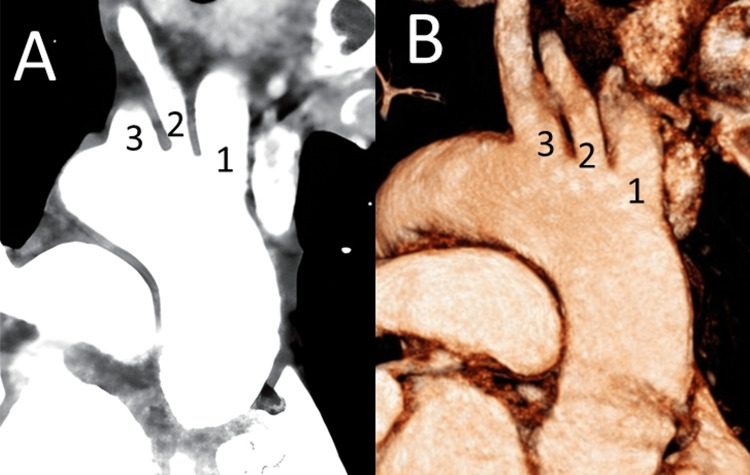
Thoracic computed tomography angiography (CTA) with focused visualization of the aortic arch and its branches, demonstrating the separate origins of the right common carotid artery (2) and right subclavian artery (3), as well as the left brachiocephalic trunk (1) arising from the aortic arch. (A) Coronal maximum intensity projection. (B) Three-dimensional volume-rendered reconstruction from a right lateral perspective.
